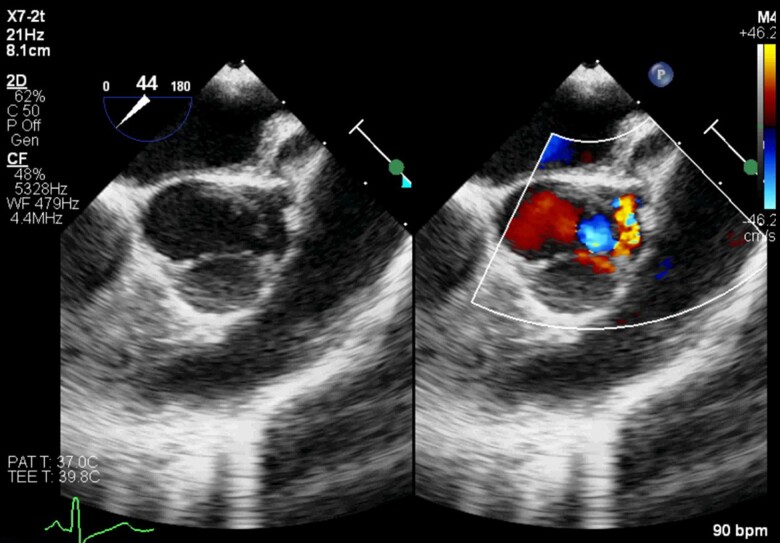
Transoesophageal echocardiogram with the aortic valve in short axis demonstrating the hypoplastic left coronary cusp (LCC), isolated coronary ostium, and obstructed coronary artery flow.
